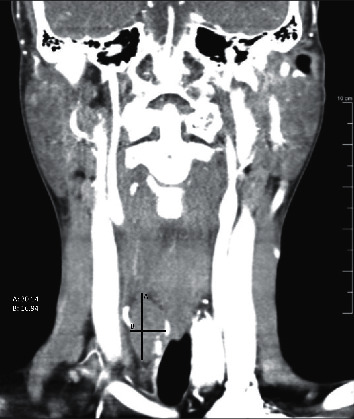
Coronal CT-neck with contrast demonstrates a 30.1 mm × 16.9 mm right-sided parathyroid adenoma causing mass effect.
